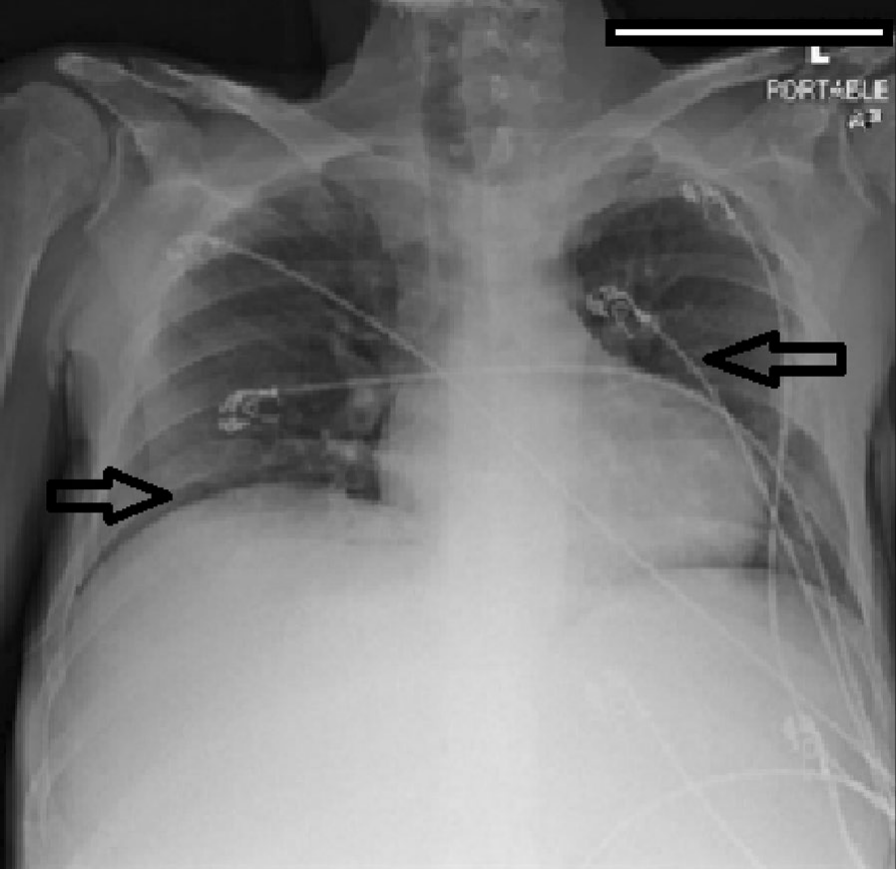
Chest x-ray showing trace bilateral pleural effusions and ill-defined erosive mass of left fourth and fifth rib
